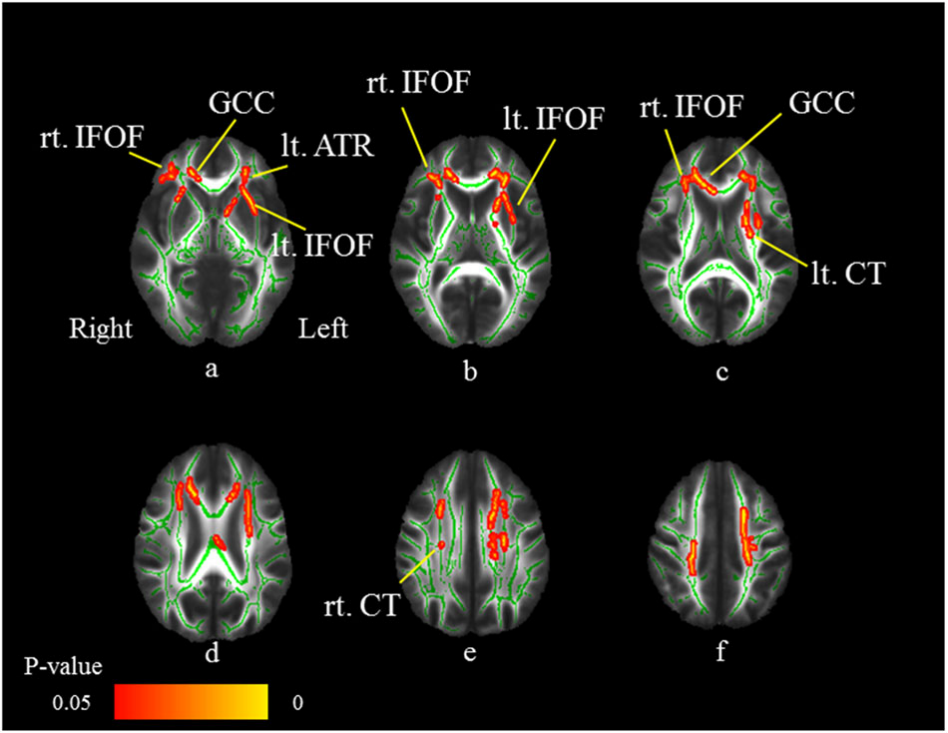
Comparison of FA findings between MDD patients and HS. Axial slices (a–f) of the cohort’s mean FA skeleton (green) overlaid with red clusters depicting significantly lower FA values in the MDD patients compared to HS (FWE-corrected *p* *<* 0.05). The MDD patients have significantly reduced FA values (FWE-corrected *p* *<* 0.05) in the right corticospinal tract (CT: e), right inferior fronto-occipital fasciculus (IFOF: a–c), left anterior thalamic radiation (ATR: a), left CT (c), left IFOF, and genu of the corpus callosum (GCC: a, c)
